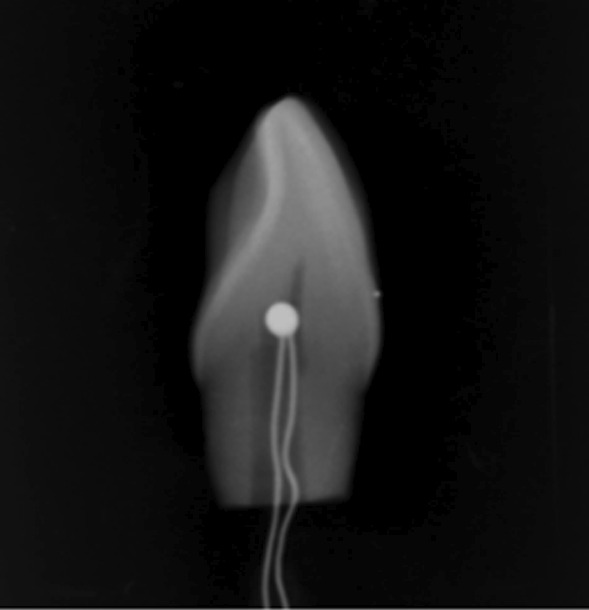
A digital radiographic image obtained to confirm the position of the thermocouple
